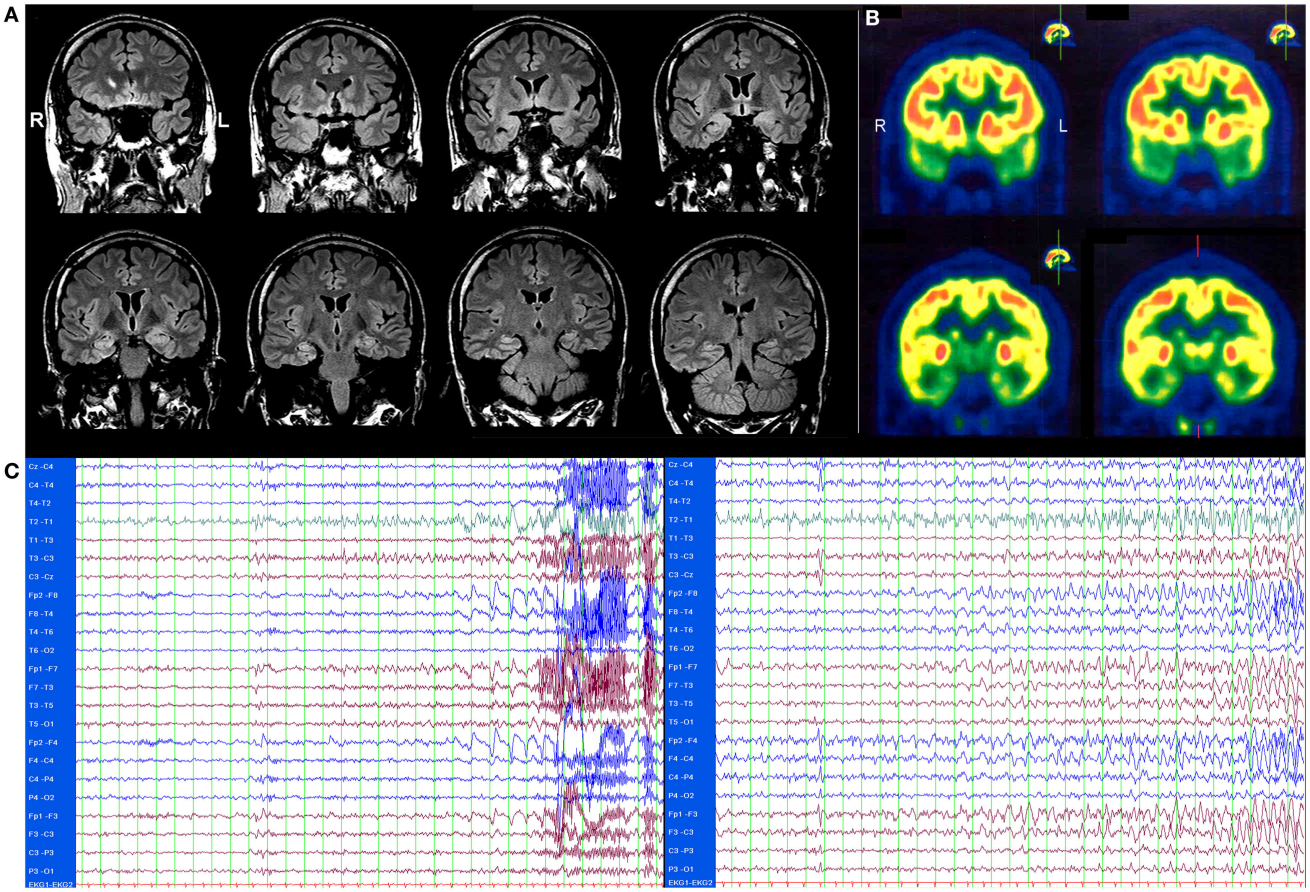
(A) Brain magnetic resonance imaging (MRI), T2/fluid attenuated inversion recovery (FLAIR) showing a distinct bilateral temporomesial hyperintensity without atrophy. (B) 2-fluoro-2-desoxy-d-glucose positron emission tomography (FDG-PET) showing a bitemporal (right > left), hypometabolism. Both images were scanned before therapy, in November 2011. (C) EEG of two seizures. One second per line. Left: seizure starting with atypical sharp-slow-wave complex and irregular theta activity left temporal. Clinical start 11 s later with eye opening, oral automatisms, leg movements, later on secondary generalization. Right: seizure starting with irregular delta activity right frontotemporal with fast transition to left frontal contacts. Clinical start 34 s later with eye opening, non-verbal vocalization, sitting up, staring, later on secondary generalization.
